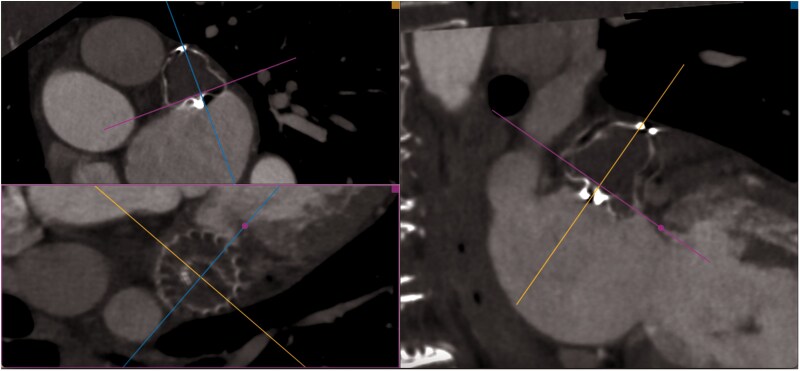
Computed tomography (CT) scan 3 months after the procedure confirmed the correct position of the device and complete LAA closure.
